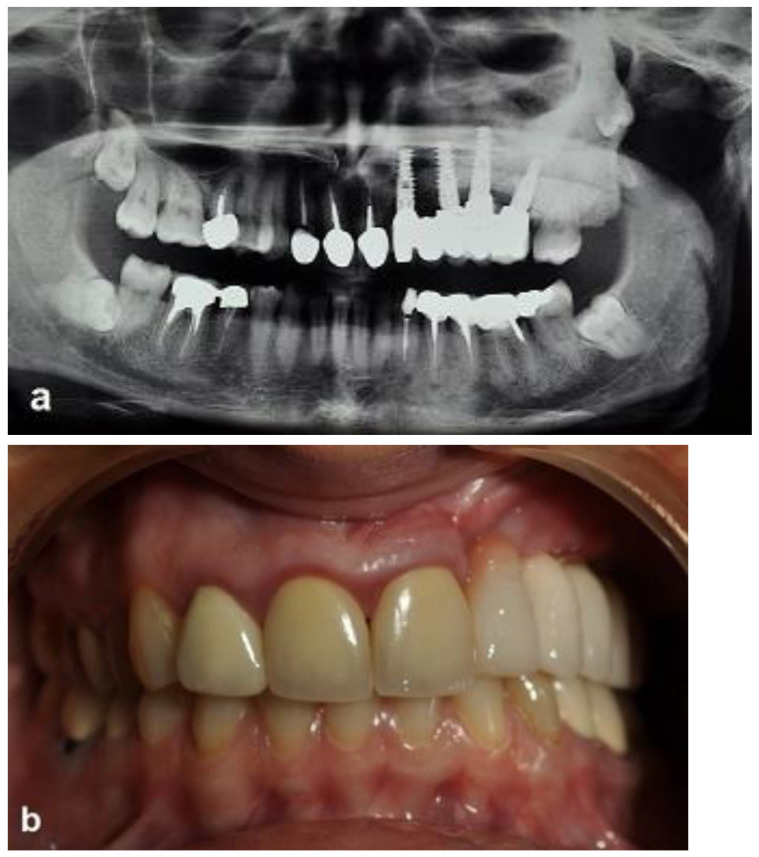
Ceramic-implant-supported fixed prosthesis one year after implant placement shows implant osseointegration and good aesthetics. (a) Radiographic view. (b) Clinical view.
